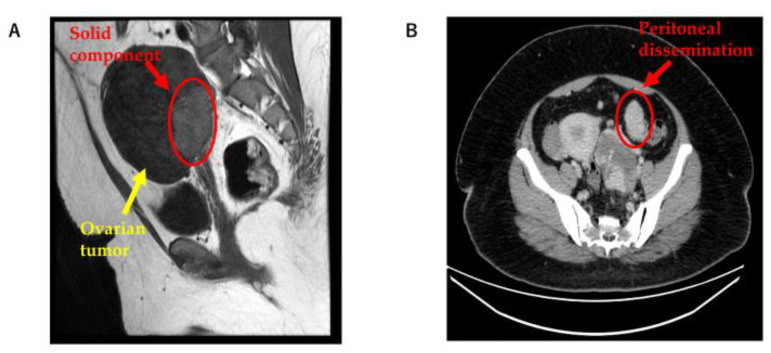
(A) MRI showing a solid component in the left ovarian tumor; and (B) CT showing peritoneal dissemination. MRI: magnetic resonance imaging; CT: computed tomography.
